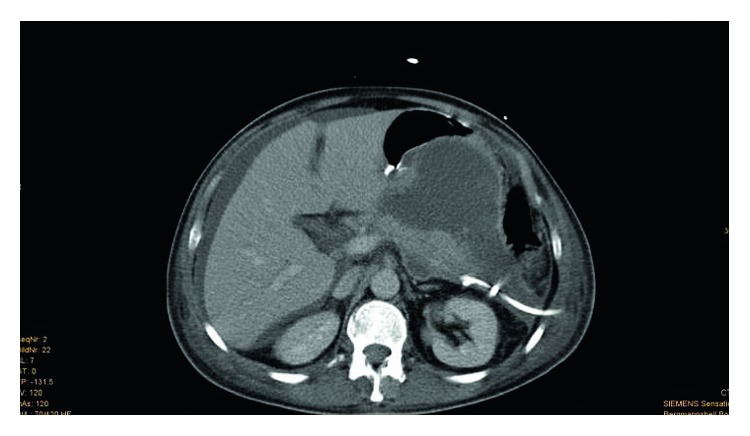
(Patient 2, scan 2) CT scan 22 days after trauma. Compared to the CT scan 7 days ago, there was an increase in septate fluid accumulation in the bursa omentalis and peripancreatically in the demarcation of the Gerota fascia. Drainage from the left side was still present. In the case of known pancreatic injury, the pancreas has a linear (sometimes up to 1 cm subtotal) interruption of the parenchyma, as in the case of subtotal rupture in the distal third of the pancreas corpus. The other parts of the pancreas were homogeneously contrasted. There was a significant increase in perihepatic ascites and no abscesses.
